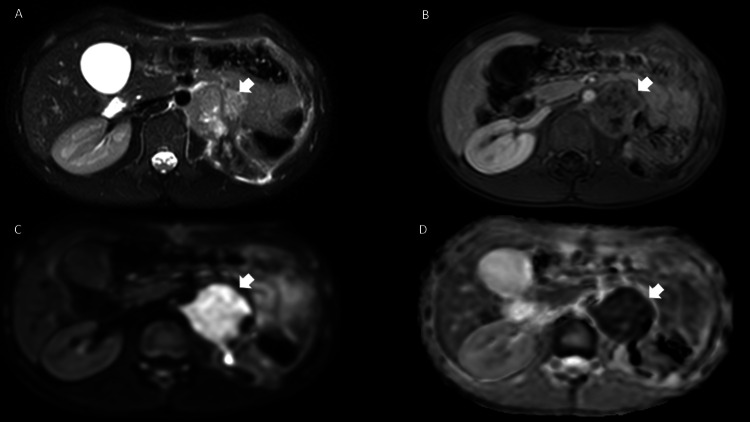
Contrast-enhanced MRI of the abdomen. Simple and contrast magnetic resonance on axial T2 sequences (A), post-contrast T1 with fat saturation (B), B800 diffusion sequence (C), and its ADC map (D). Changes due to left nephrectomy, with the persistence of retroperitoneal lymphadenopathies. (arrows in A, B, C and D).
